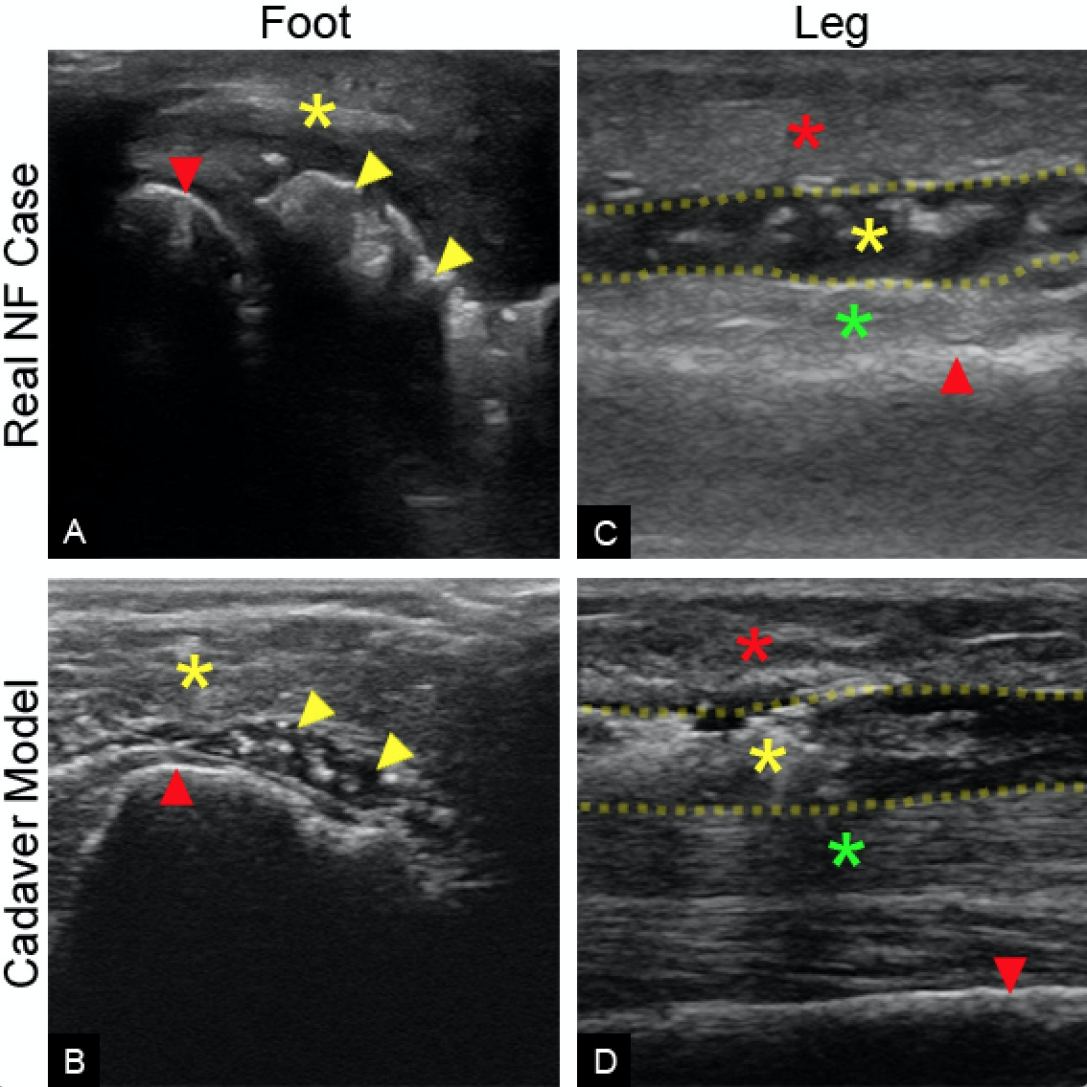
Sonographic Comparisons Ultrasound comparison of the cadaver model and real cases of NF in the foot and leg showing a very similar sonographic appearance. A) Long-axis ultrasound of a real case of NF in the foot showing gas collection (yellow arrowheads) distal to the calcaneus (red arrowhead) and deep to the subcutaneous tissue (yellow asterisk). B) Long-axis ultrasound of the cadaver model of the foot showing gas and fluid collection (yellow arrowheads) distal to the calcaneus (red arrowhead) and deep to the subcutaneous tissue (yellow asterisk). C) Sagittal ultrasound of a real case of NF in the leg showing subcutaneous tissue (red asterisk), fluid collection (yellow asterisk) within crural fascia (yellow dotted line), tibialis anterior (green asterisk), and tibia (red arrowhead). D) Sagittal ultrasound of the cadaver model of the leg showing subcutaneous tissue (red asterisk), fluid collection (yellow asterisk) within crural fascia (yellow dotted line), tibialis anterior (green asterisk), and tibia (red arrowhead).
